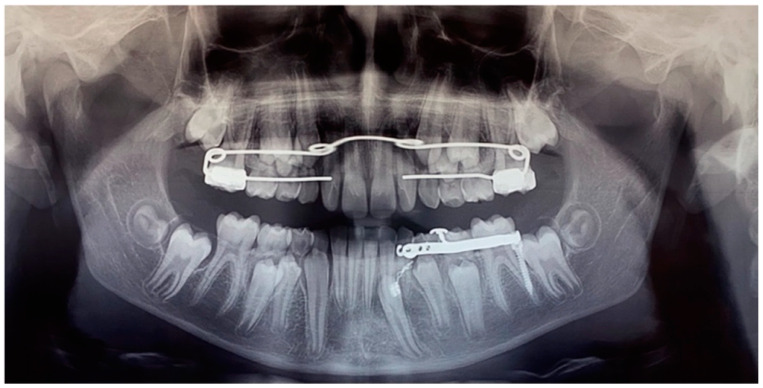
Panoramic radiography of treatment progress (1 year after the first panoramic radiograph).
